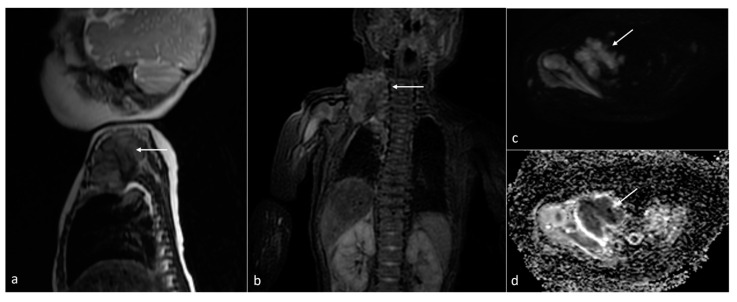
Malignant rhabdoid tumor (MRT) of the brachial plexus (arrows) with infiltration of the right clavicle, right lung, and muscles in a newborn girl. Inhomogeneous mass with low ADC values. (a) Sagittal T2WI; (b) coronal STIR; (c) axial DWI; (d) corresponding ADC map.
